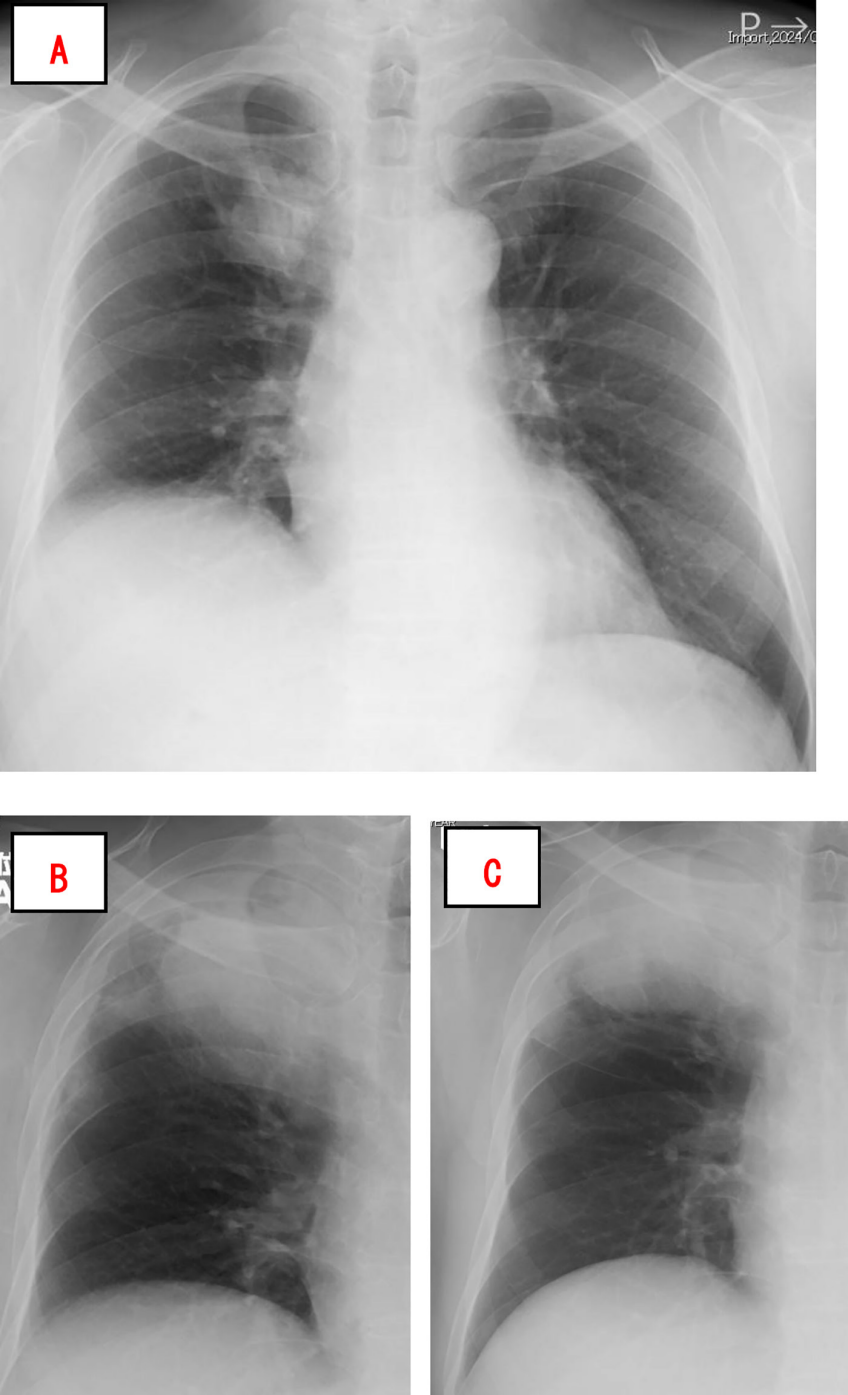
Imaging findings. Chest radiography performed at the initial visit revealed a mass in the right upper lung field (A). Chest radiograph on admission, 1 month after the initial radiograph, showing enlargement of the mass in the right upper lung field (B). Chest radiograph obtained 14 days after antibiotic therapy showing no improvement in the imaging findings (C).
